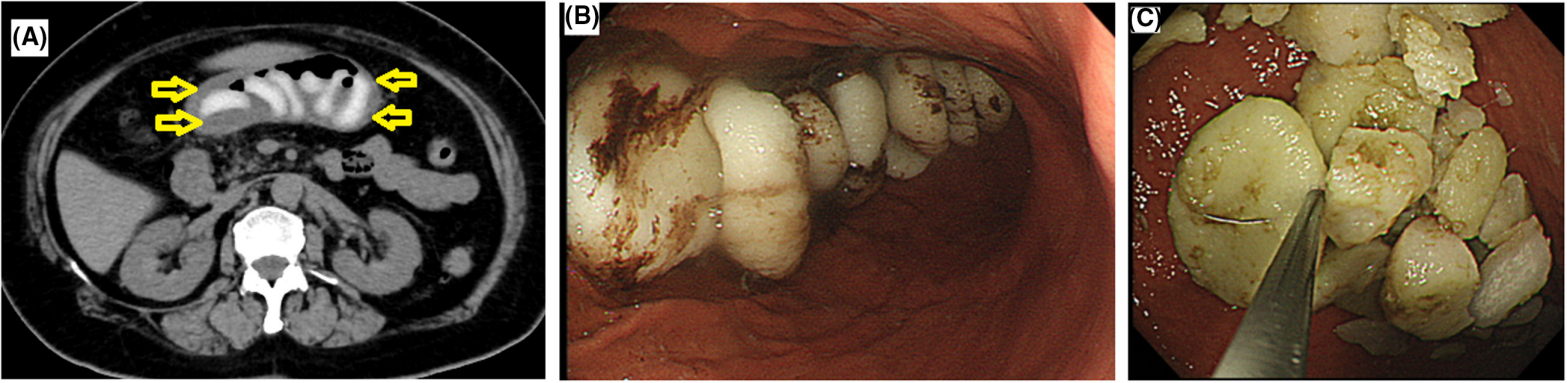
(A) CT revealed multilayer arc‐like high‐density objects in the stomach. (B) Endoscopy revealed 10 completely intact round mochi. (C) We endoscopically sliced the mochi to <10 mm by snaring without electrical current.
